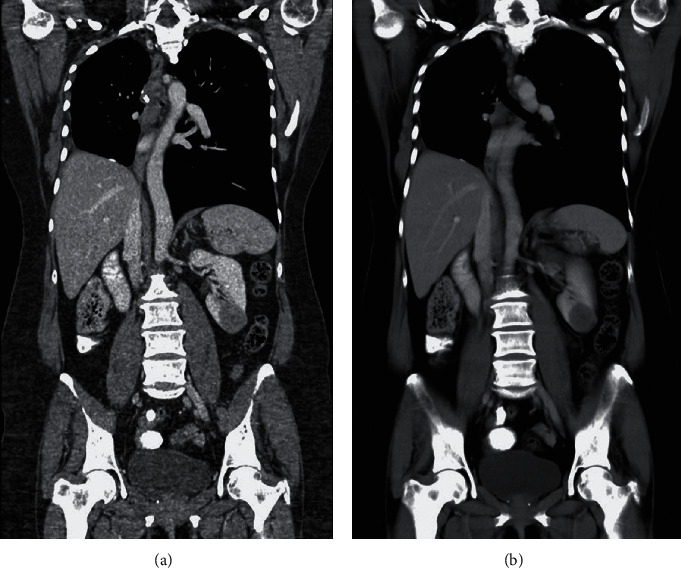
Contrast-enhanced computed tomography in arterial (a) and venous (b) phases, revealing a slightly enhancing mass occupying the lower pole of the left kidney.
